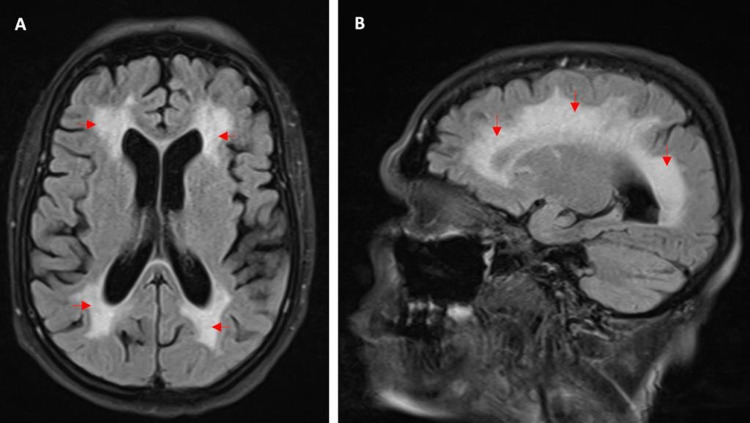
Brain MRI showing extensive chronic microangiopathic changes (A) Axial and (B) sagittal views of the brain (fluid-attenuated inversion recovery (FLAIR) sequence) showing confluent T2 high signal intensity involving the periventricular, deep and subcortical white matter, suggestive of extensive chronic microangiopathy.
